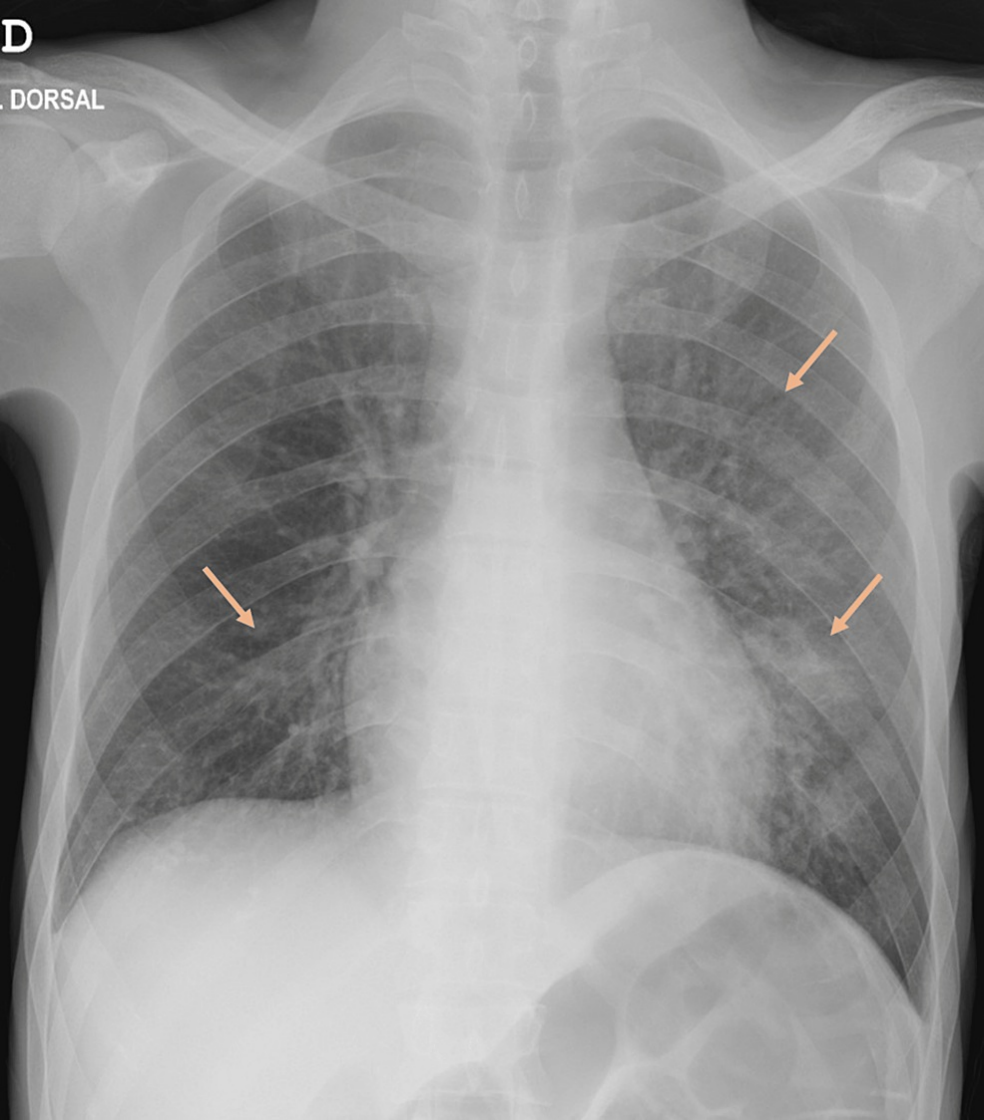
Chest X-ray showing bilateral interstitial infiltrates (arrows).
